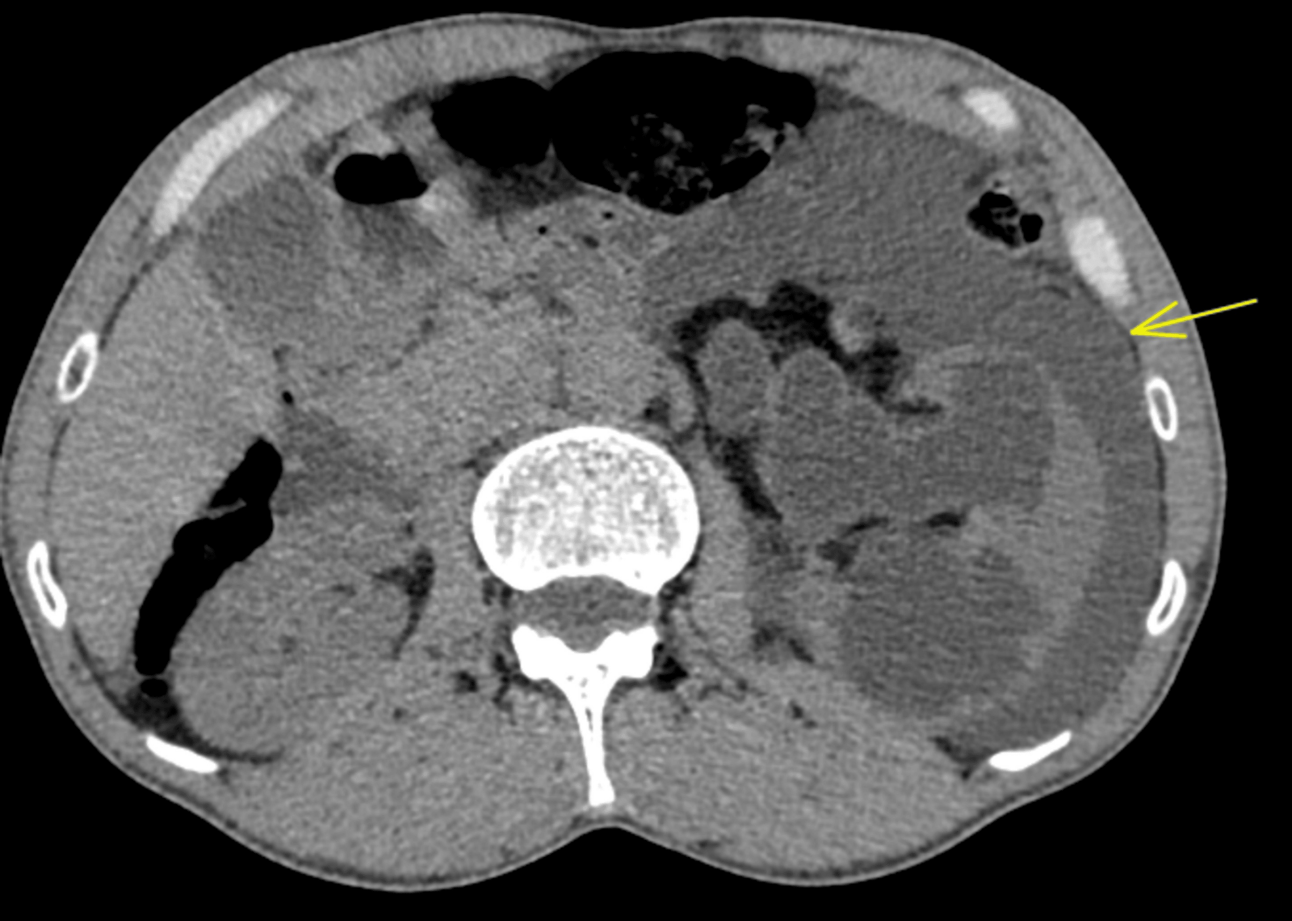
Axial section of a CT of the abdomen revealing a significant left pyelo-ureteral dilatation with perinephric collection (yellow arrow).
